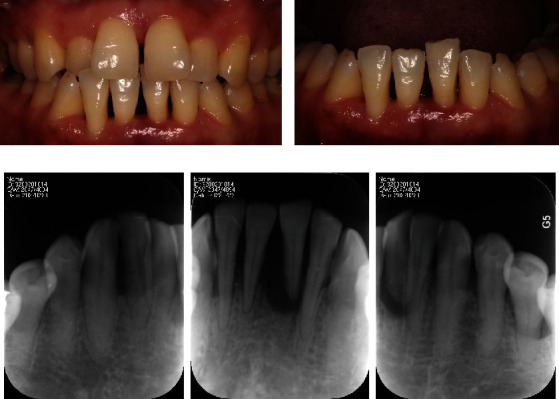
Intraoral photograph before the extraction of the mandibular left central incisor. (a) Labial view of anterior teeth. (b) Protrusion of the mandibular left central incisor. (c) Periapical film of the mandibular anterior teeth.
